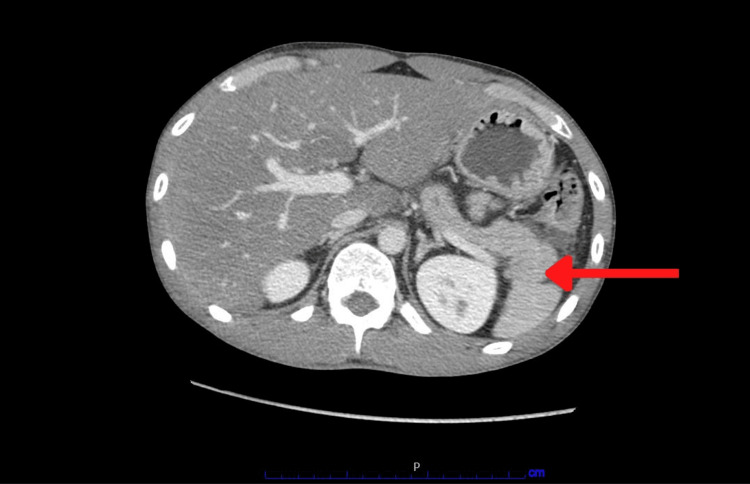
Computed tomogram of the abdomen and pelvis showing acutely inflamed pancreatic parenchyma.
